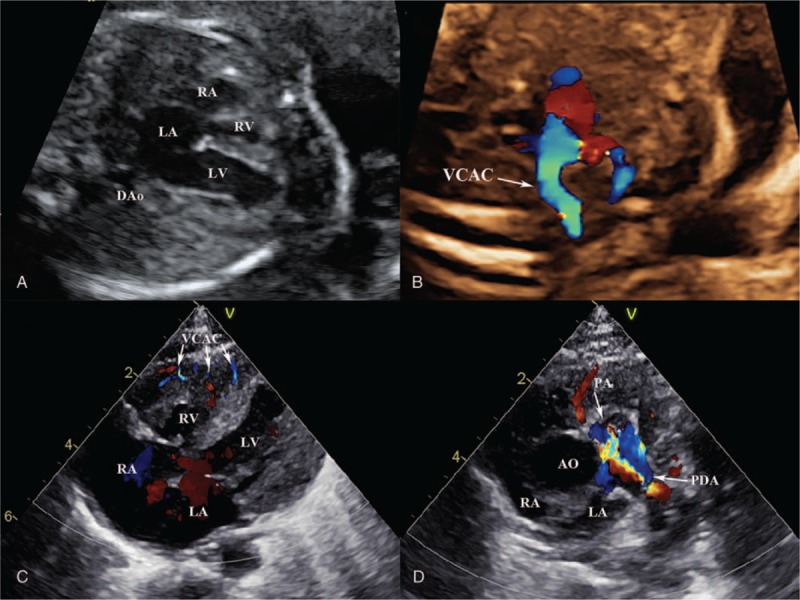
The prenatal and postnatal echocardiography of type III pulmonary atresia with intact ventricular septum. (A) The prenatal echocardiography at 25 weeks’ gestation, four-chamber view shows the absence of right ventricular trabecular portion, intact ventricular septum. (B) Color Doppler shows venticulo-coronary artery communication (*narrow*). (C) The postnatal echocardiography of the same fetus, four-chamber view shows the absence of right ventricular trabecular portion, venticulo-coronary artery communication (*narrow*). (D) Parasternal short axis shows pulmonary atresia (*arrow*), patent ductus arteriosus. DAo = descending aorta, LA = left atrium, LV = left ventricle, PA = pulmonary atresia, PDA = patent ductus arteriosus, RA = right atrium, RV = right ventricle, VCAC = ventriculo-coronary artery communication.
